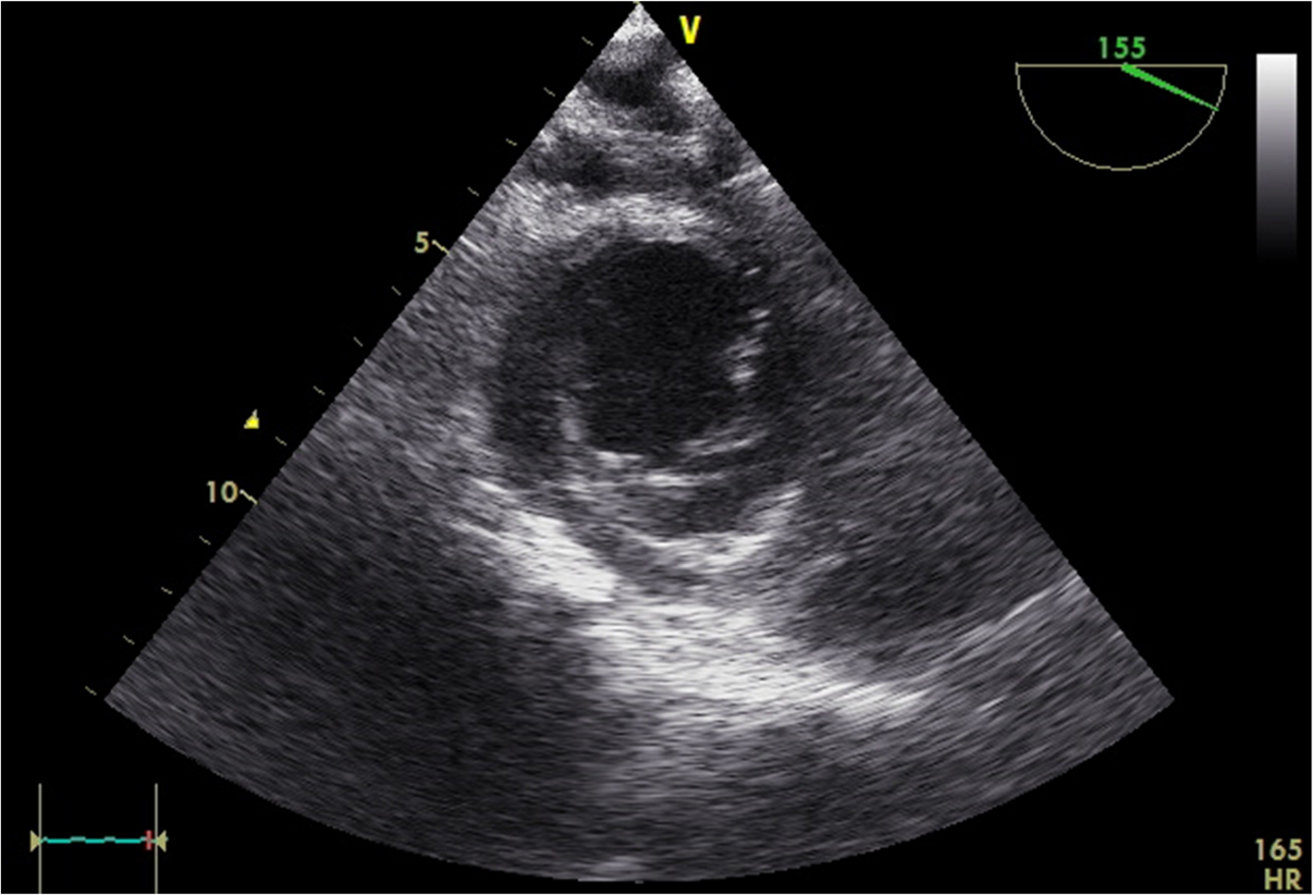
A representative transgastric short axis transesophageal view. Such a view is useful for assessing early regional wall abnormalities indicative of myocardial dysfunction and overall volume status
